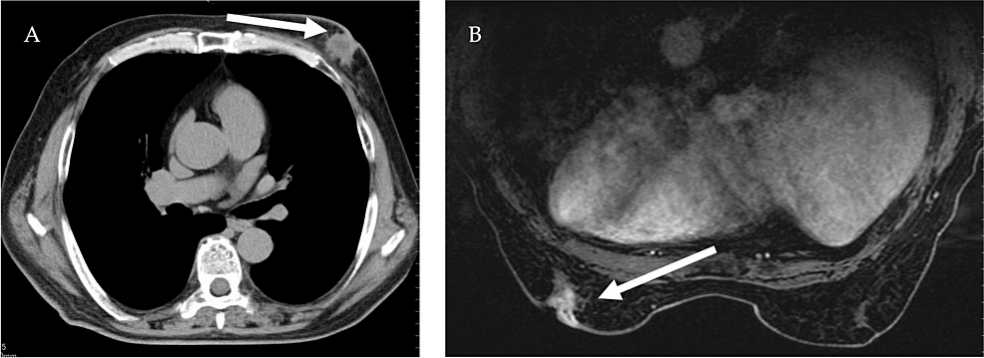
Chest CT findings and contrast-enhanced mammography MRI findings (T2-weighted imaging) (A) CT scan of the chest showed an irregular nodular structure under the left nipple with a low absorptive area inside (arrow). (B) MRI with contrast-enhanced mammography showed an irregular nodule under the left nipple with a strong enhancement effect and a marginal predominance (arrow). The enhancement effect was negative for breast cancer.
